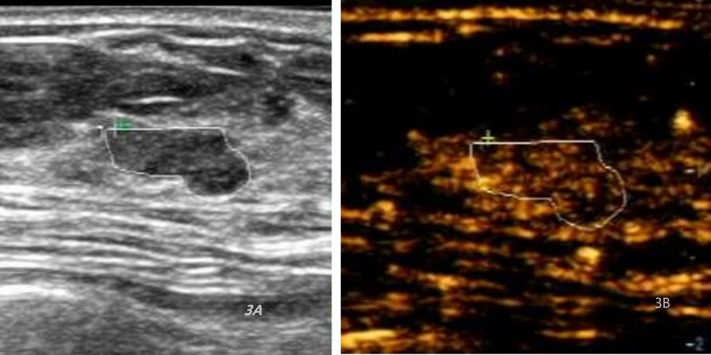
Ultrasound and CEUS images of a 40-year-old female patient with adenosis. (A) Ultrasound (US) image indicating a hypoechoic lesion with clear boundaries and irregular morphology, resulting in a total score of 6. (B) Contrast-enhanced ultrasound (CEUS) image depicting homogeneous equal enhancement, indistinct enhancement borders,resulting in a total score of 6.
